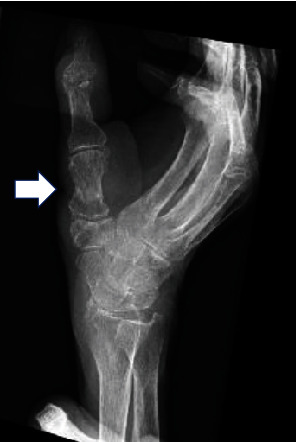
Bone union of the first metacarpal bone (arrow) is visible on a radiograph taken 1 year after the injury.
